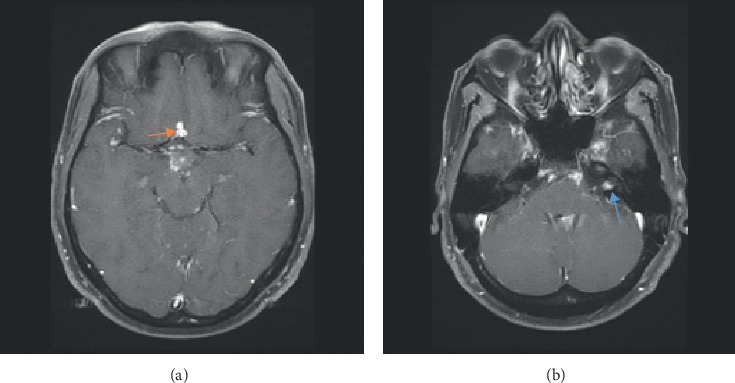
(a) MRI brain, axial depicting nodular enhancement, a low frontal lesion close to the anterior communicating artery lobe (orange arrow). (b) Nodular enhancement of the left internal auditory canal (blue arrow).
